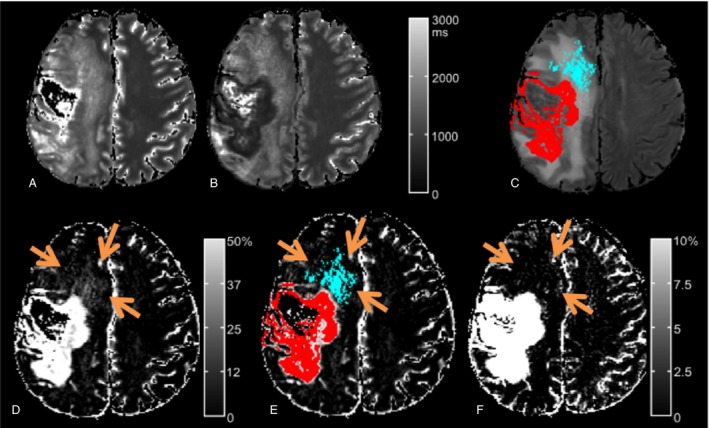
Quantitative T1 map of patient N 3 (male, age 67). T1 maps pre (A) versus post (B) contrast agent show the T1‐shortening due to the impaired BBB induced by the tumor. The subtraction map ∆qT1 (D) not only delineates the solid‐enhancing tumor compartment (red in C and D), but also areas with cloudy enhancement in the vicinity of the solid tumor (blue in C and D) that is inside, but not congruent with the FLAIR hyperintensity (C). This cloudy‐enhancing compartment is not visible on conventional T1‐weighted subtraction images (F) regardless of the window width chosen for display. Even more, at a low threshold window (note scale in F), noise becomes visible in the whole white matter and in the necrosis. The semiautomated volumetry defined cloudy‐enhancing compartment (blue) by a T1‐decrease of 10–50% and solid‐enhancing compartment (red) by T1‐decrease of more than 50% of T1‐decrease postcontrast. FLAIR, Fluid‐attenuated inversion recovery.
